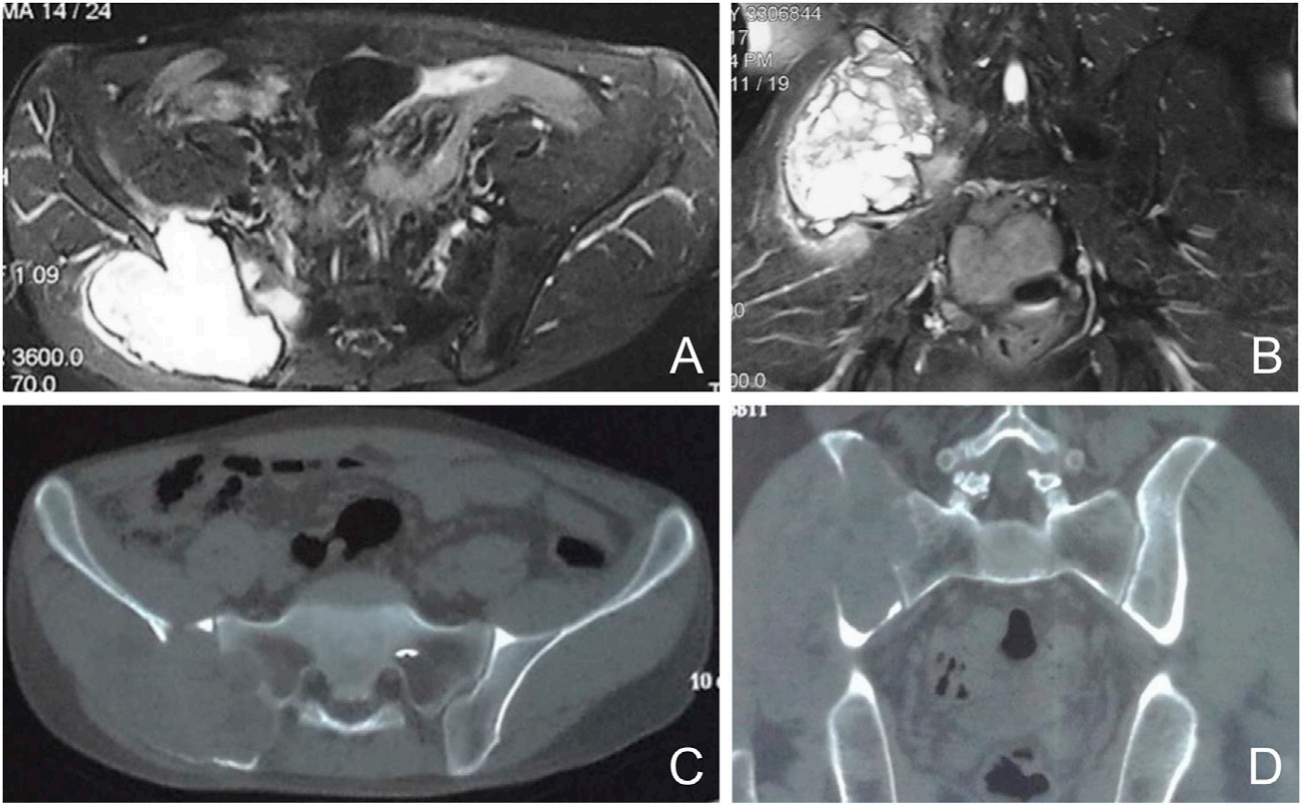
Preoperative imaging of a representative case. Axial and coronal nuclear magnetic resonance images show a cumulative lesion of the sacroiliac joint with edema (A, B). Axial and coronal computed tomography images show a cumulative osteolytic lesion of the sacroiliac joint (C, D).
